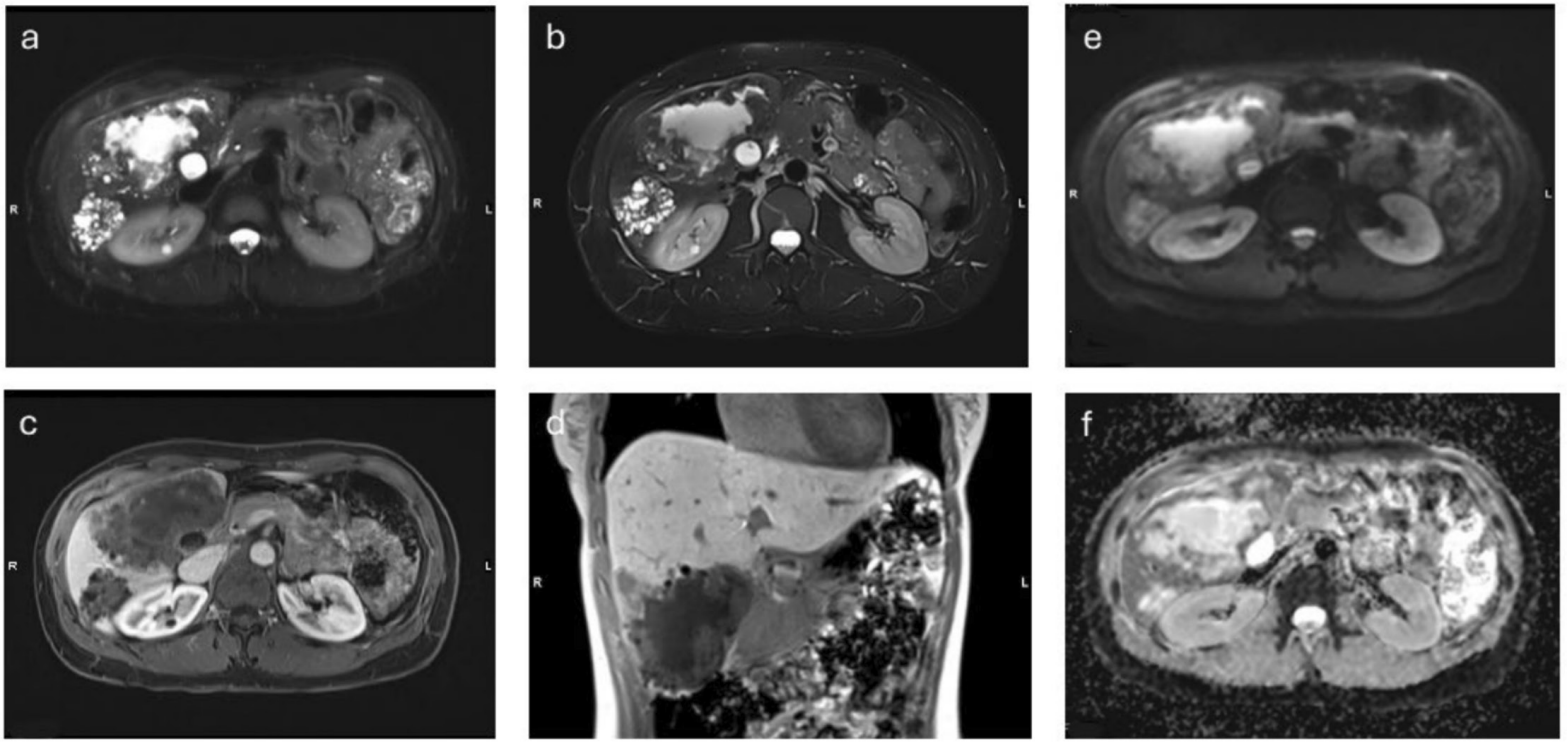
MRI of a 46‐year‐old female with a palpable mass of the upper right abdomen showing inhomogeneous liver parenchyma with multiple cystic lesions in segments 4a/b and 5–7 and multiple smallnodular foci. (a) Axial, noncontrast conventional HASTE sequence; (b) axial, noncontrast DL‐HASTE sequence; (c) contrast‐enhanced T1‐sequence (venous phase); (d) coronal contrast‐enhanced T1 (venous phase); (e) diffusion map (TRACE); and (f) diffusion map (ADC).
